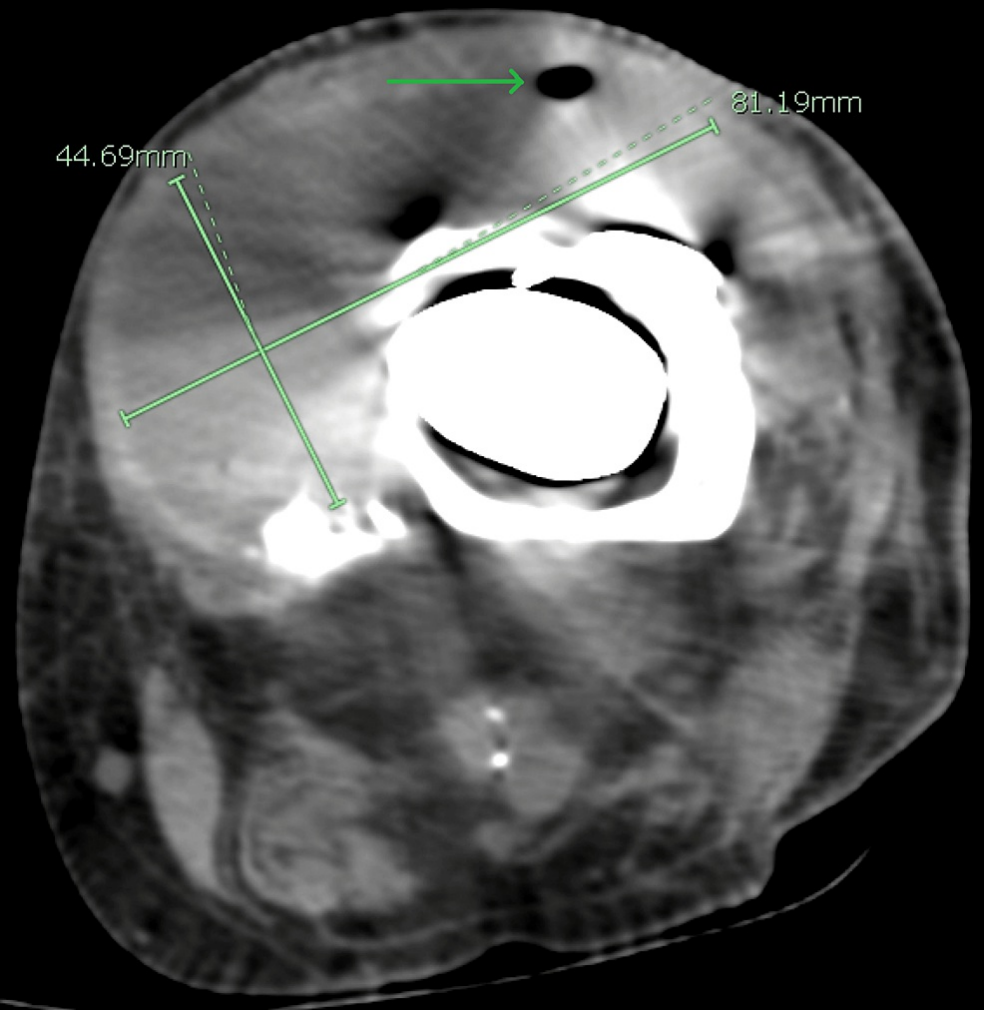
CT of the left lower extremity, transverse cut, showing gas bubbles (green arrow) in the fluid collection, suggestive of an abscess. CT, computed tomography
